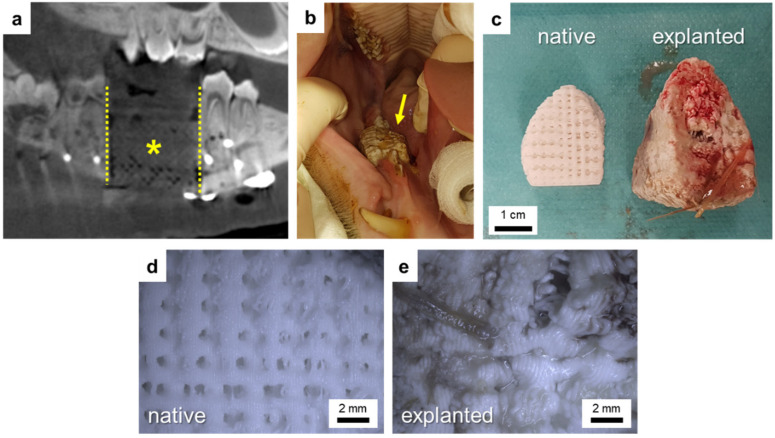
Minipig #1: good fit of the scaffold into the segmental mandibular defect as verified by X-ray analysis directly after implantation (a) (dotted lines = osteotomy borders, * = scaffold); examination at 6 weeks postoperation showed a large intraoral dehiscence (b) (arrow = dehiscence/swollen scaffold) due to a swollen scaffold (c–e).
